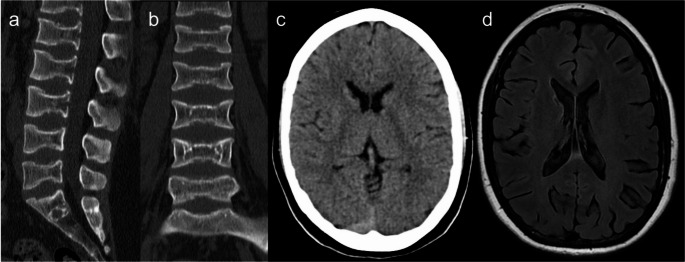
Spondyloenchondrodysplasia with immune dysregulation (SPENCDI). A 19-year-old female patient with short stature, paraparesis, and systemic lupus erythematosus. (a and b) Lumbar spine CT scan shows platyspondyly. (c) No intracranial calcifications are observed on head CT scan. (d) FLAIR images reveal a small cavitated lesion in the head of the right caudate nucleus with surrounding gliosis, likely of unrelated vascular etiology
